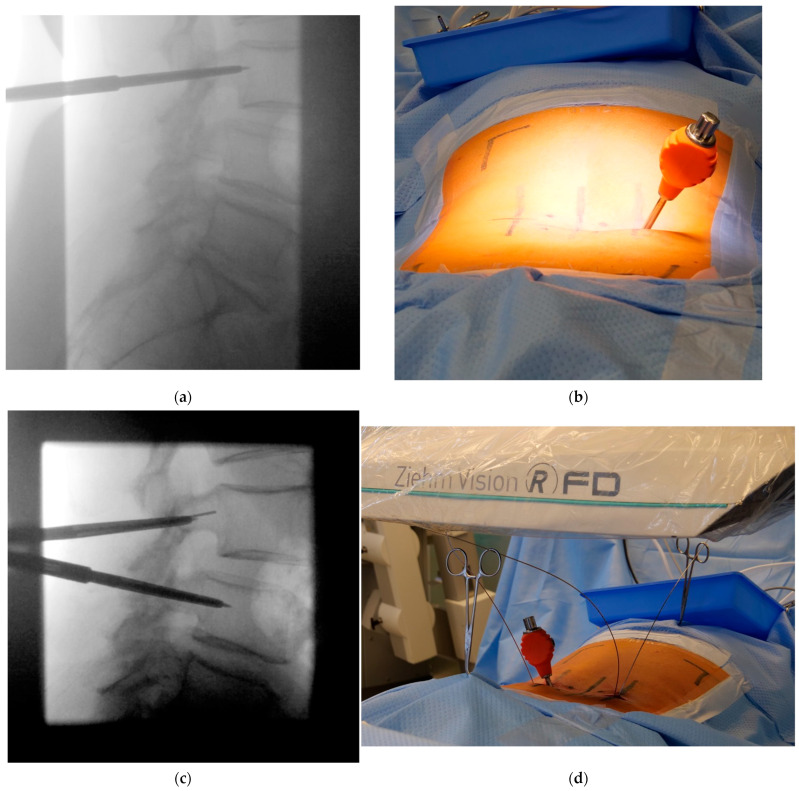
(a) Pediculation with the Jamshidi using tactile feedback under sparse lateral radiographic control followed by K-wire insertion. (b) The corresponding convergence angle must be followed. It is not necessary to hold the instruments while performing the fluoroscopic imaging. (c) To save on fluoroscopic images, Jamshidi needles can be inserted on both sides simultaneously—preferably into different vertebral bodies to allow for radiographic differentiation. (d) The Jamshidi needle does not have to be held during fluoroscopic imaging; thus, standing behind a mobile radiation protection wall is possible.
